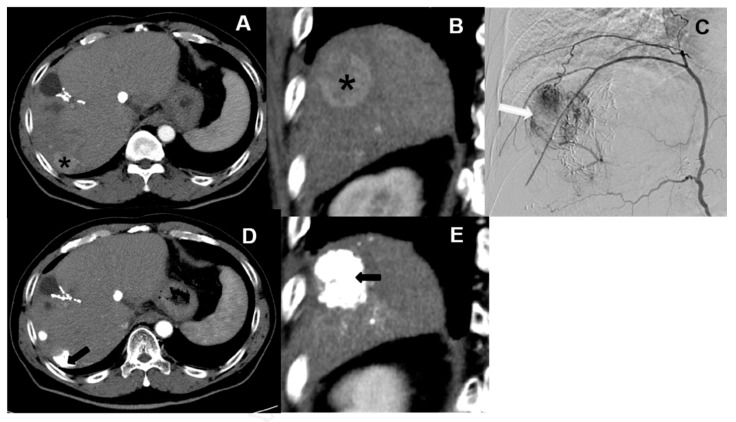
Traditional embolization (cTACE). Hepatocellular carcinomas (HCC) with arterial enhancement in segment 7 of the liver in both CT images (A and B, * star) and angiography (C, white arrows). TACE is performed by using conventional TACE with lipiodol accumulation (D and E, black arrows) on follow up CT.
